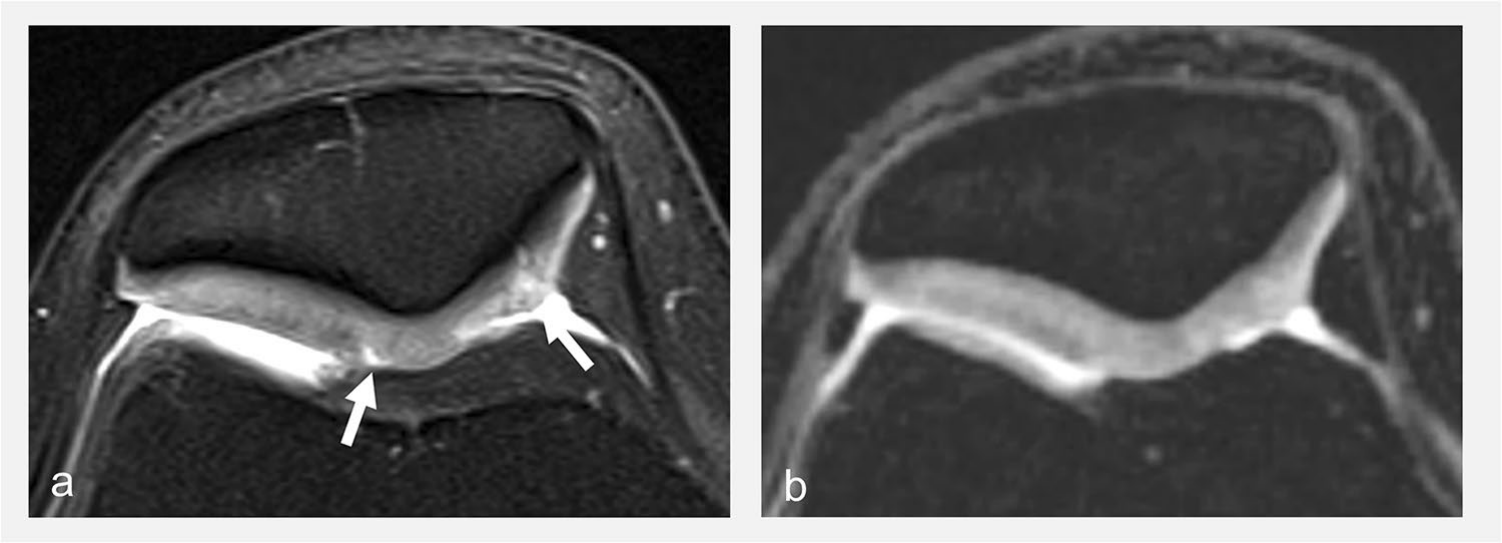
Transverse FS IW (a) and transverse reformat of 3D-double echo steady state (DESS) (b) MRI sequences obtained on a 3T MRI unit at the level of the patellofemoral joint. Cartilage fissures and intra-chondral high signal abnormalities are well visualized on FS IW image (arrows in a) but are occult on 3D-DESS
